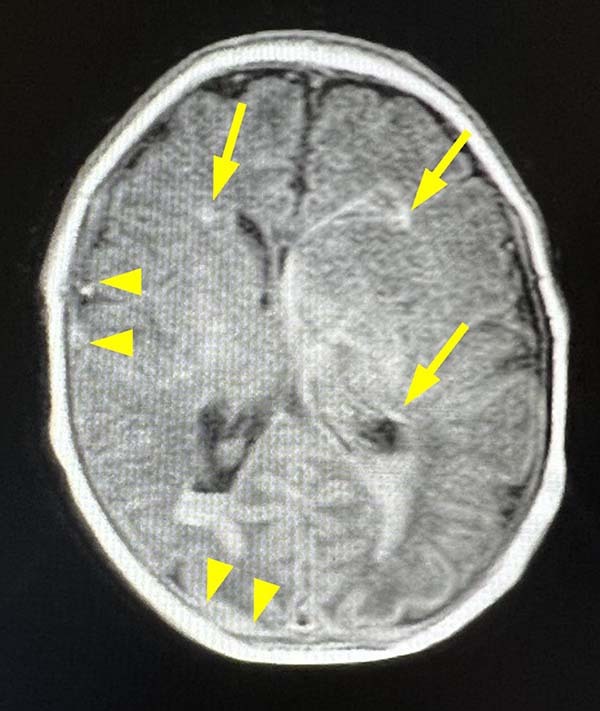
Magnetic resonance imaging (MRI) scan of the brain. Axial view, T1-weighted images show multiple parenchymal hemorrhages (arrows) and subdural hemorrhages (arrowheads).
